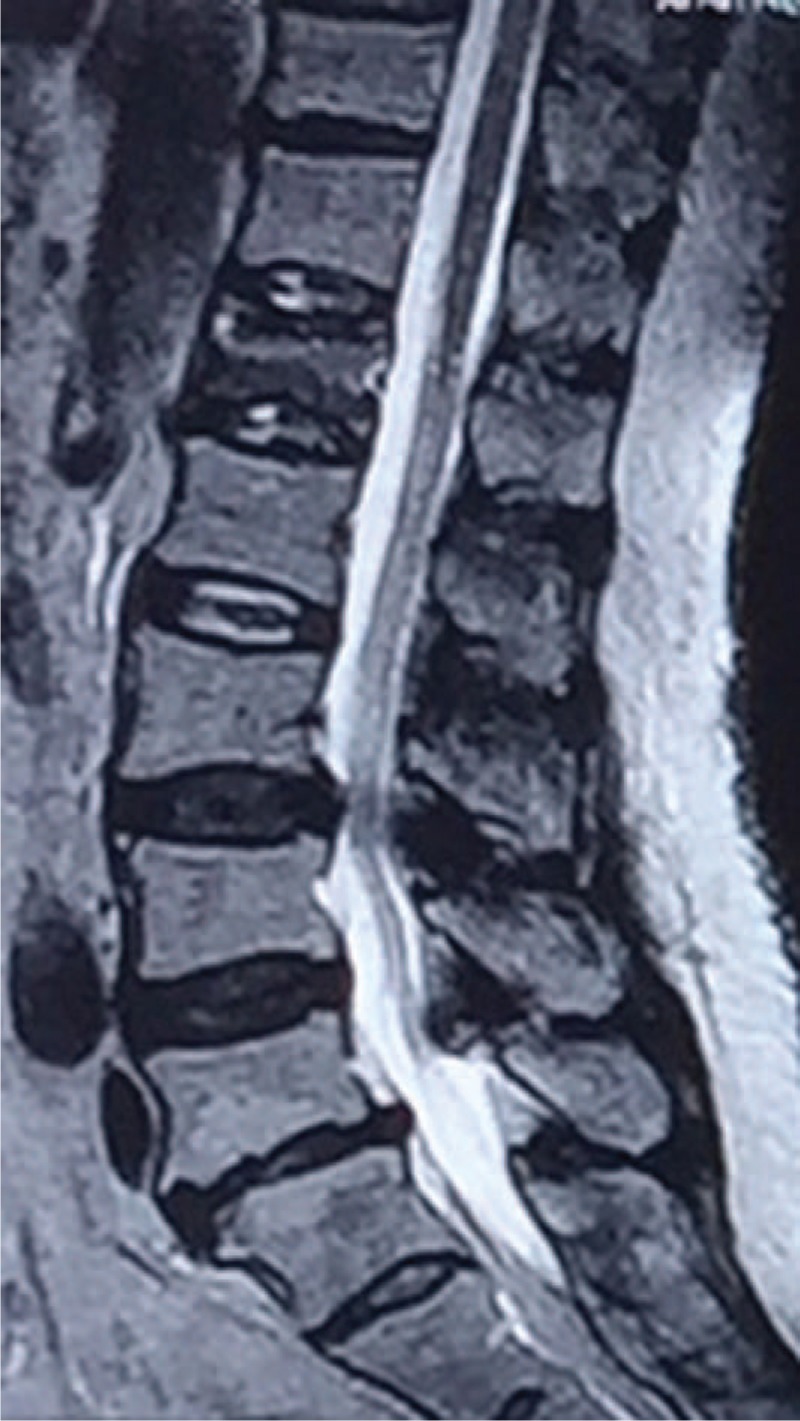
T2-weighted sagittal magnetic resonance imaging (MRI) of the lumbar spine showed an acute osteoporotic L1 compression fracture. MRI = magnetic resonance imaging.
